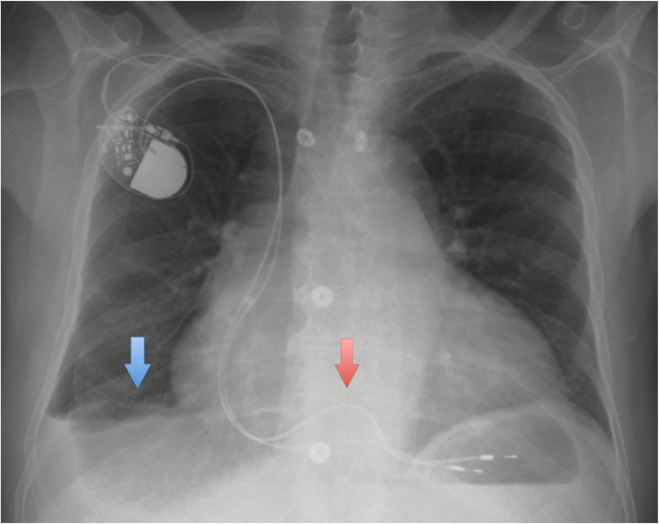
Preoperative chest X-ray. Blue arrow: pleural effusion; Red arrow: different course of the two leads through the tricuspid valve.
